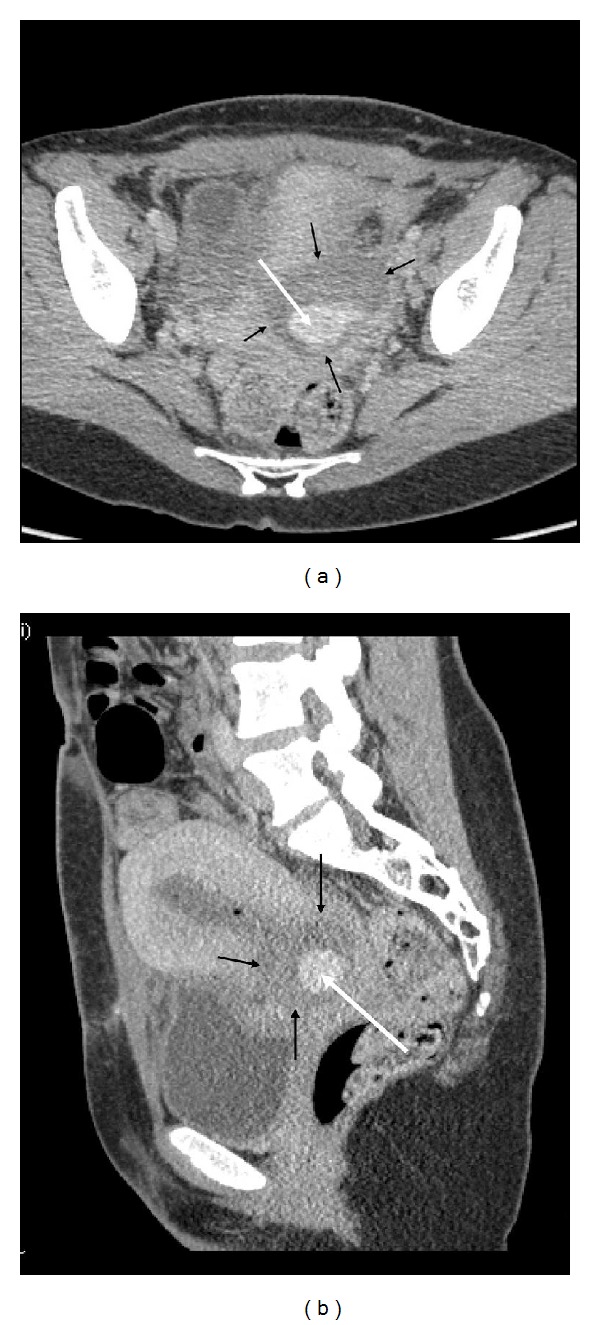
CT angiography of pelvis with axial (a) and sagittal (b) images. The large hematoma is located at the left part of pelvis in the wall of cervix (black arrows). The white contrast shows the leakage into the hematoma (white arrow).
